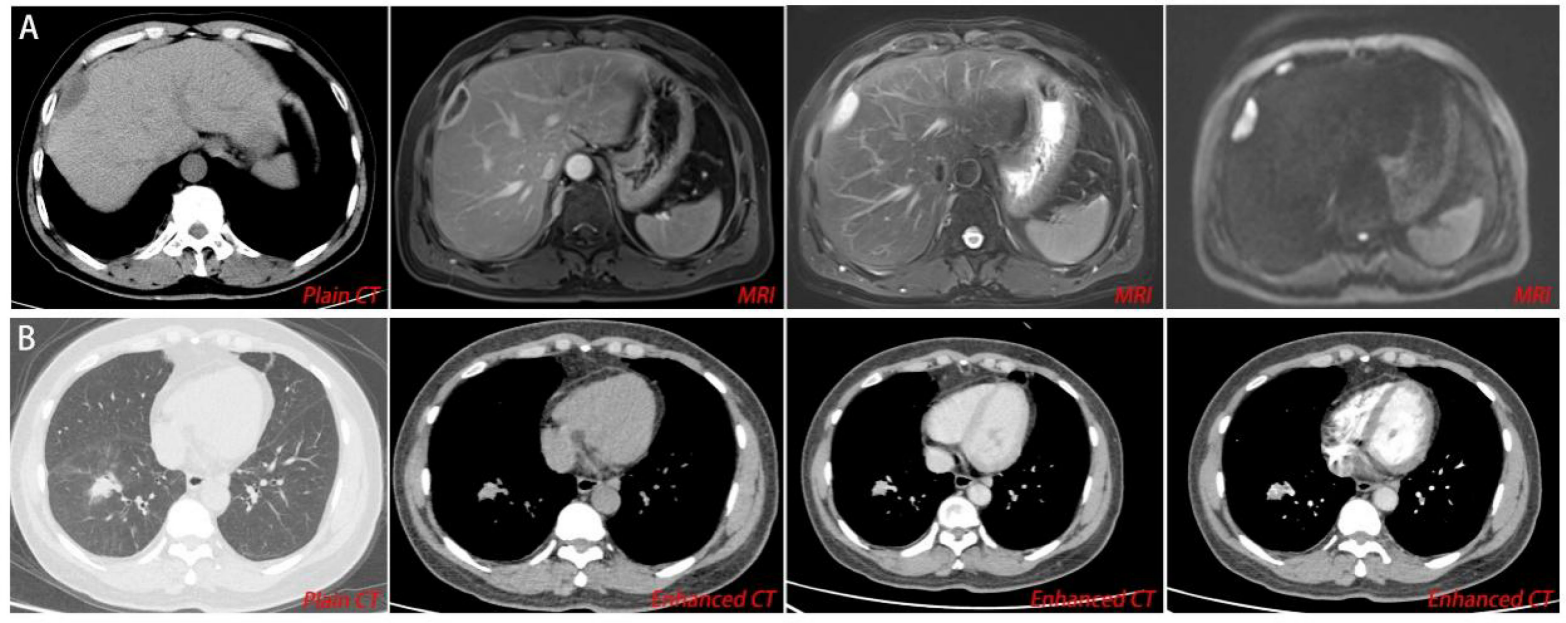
Imaging examination. (A) For hepatic tuberculosis, CT and MRI showed ring-shaped delayed enhancement during the arterial, venous, and delayed phases. (B) For pulmonary tuberculosis, enhanced CT scans showed uneven enhancement, moderate blood flow perfusion, adjacent bronchial stenosis, compression displacement, and vascular shadows passing through.
